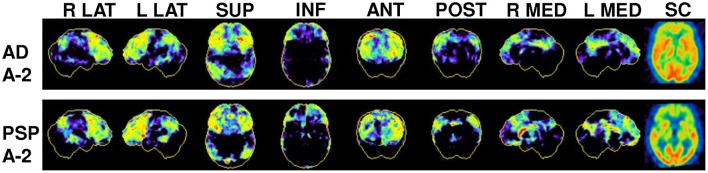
Examples of conflicting FDG-PET findings in two patients with suspected PSP: Representative 3D-SSP for FDG-PET from right/left lateral (R LAT/L LAT), superior (SUP), inferior (INF), anterior (ANT), posterior (POST), right/left medial (R MED/L MED) and a subcortical (SC) horizontal plane image of SUV for comparison of typical AD and PSP metabolic patterns. Note that both patients showed a frontal pronounced hypometabolism but as well an AD-like hypometabolism in the posterior cingulate cortex and in parietal cortices. Clinical follow-up identified one patient as AD and one as PSP.
